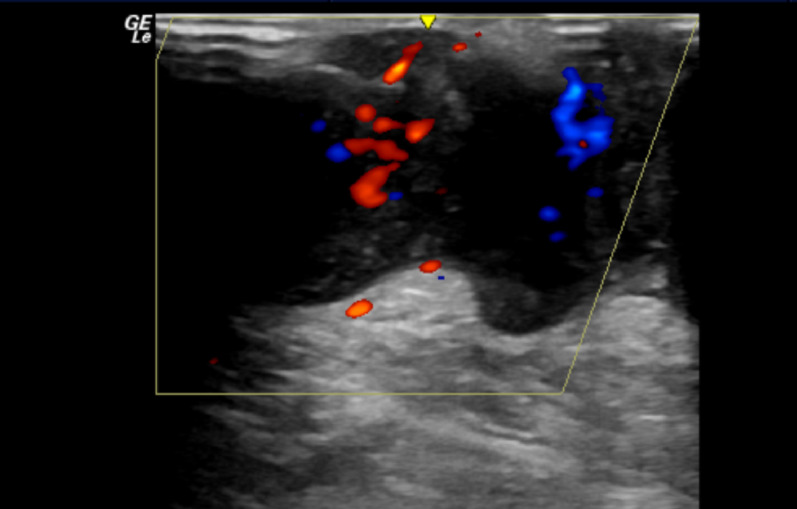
Ultrasound show intraluminal solid mass with both venous and arterial flow signals
